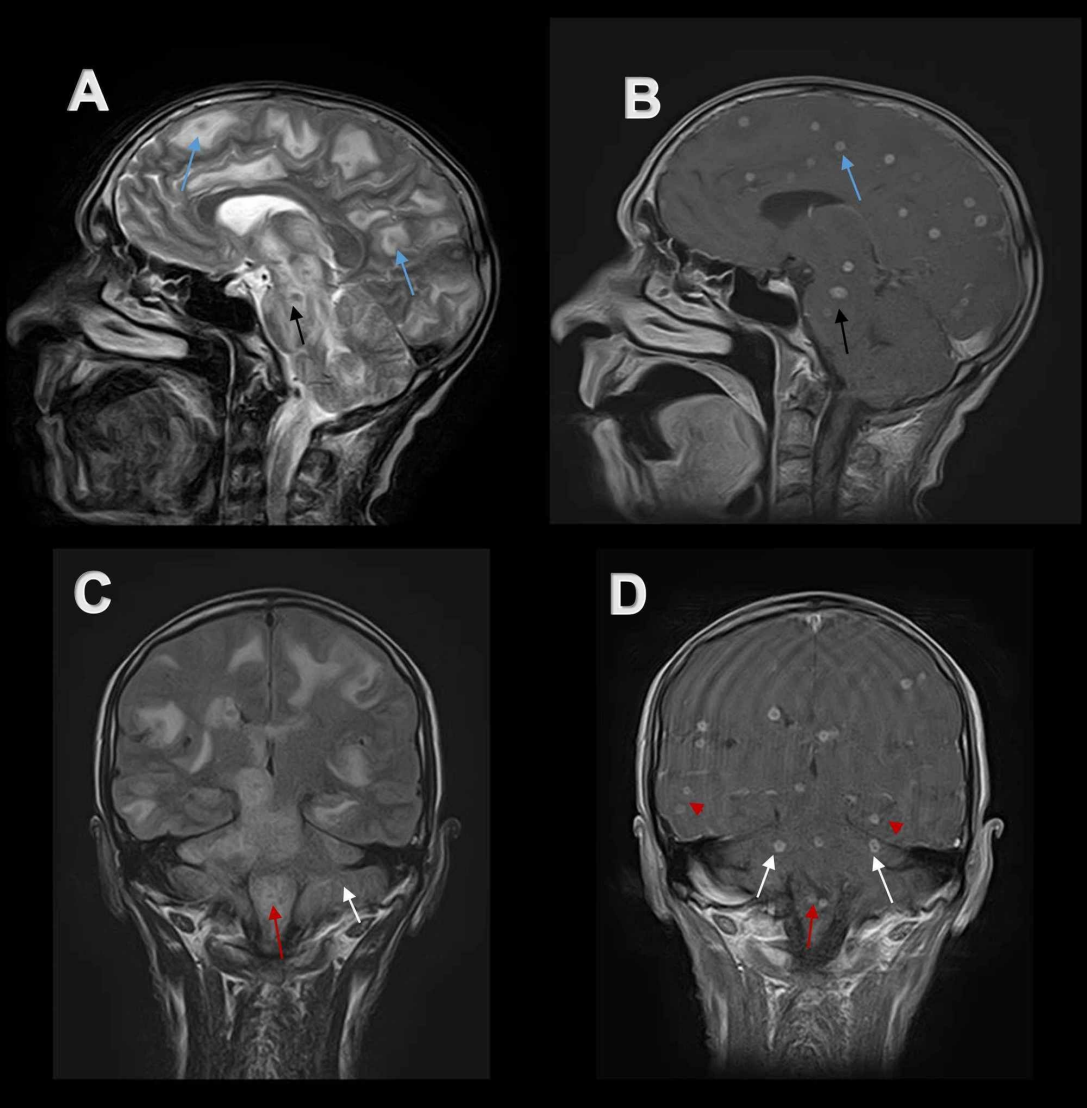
Sagittal and coronal MR imaging of the brain showing the extent of involvement (A) Sagittal T2-weighted MR image and (B) post-contrast sagittal T1-weighted MR image exhibit numerous lesions in upper brainstem (black arrows) and cerebral hemisphere (blue arrows). (C) Coronal FLAIR MR image and (D) post-contrast T1-weighted coronal MR image show widespread lesions in the lower brain stem (red arrows), bilateral temporal lobes (red arrow heads), and cerebellum (white arrows). MR, magnetic resonance. FLAIR, fluid-attenuated inversion recovery
